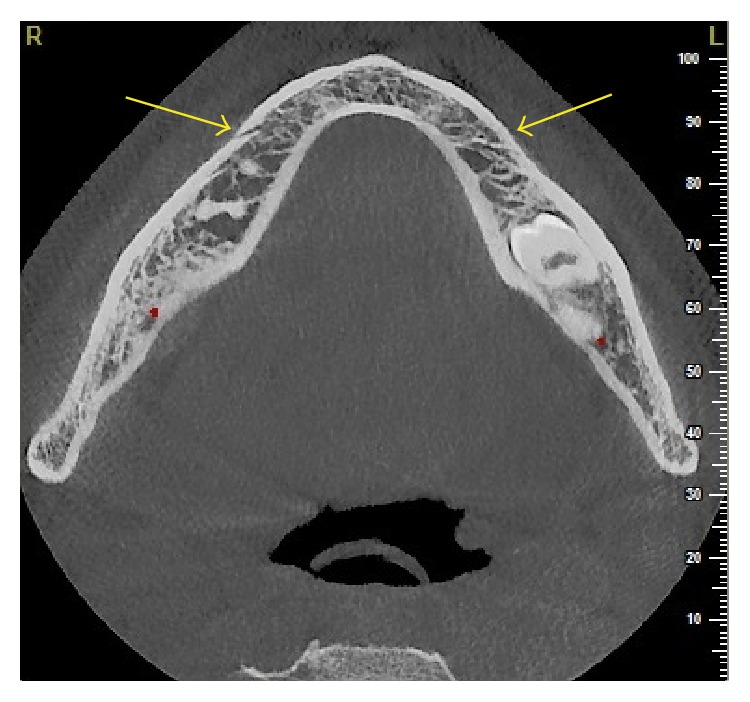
Transverse CBCT slice of the right MF is pointed by the arrow and the left MF area protuberance is pointed by the arrow.
